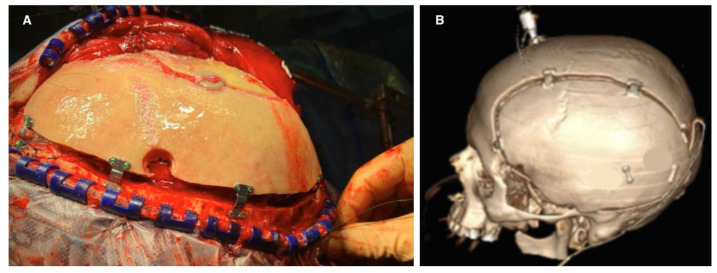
Panel (A) shows an intraoperative view of an expansion craniotomy with specialized fixation plates in place, demonstrating elevation of the bone flap above the cranial rim. Panel (B) presents a postoperative three-dimensional CT reconstruction confirming the maintained elevation of the bone flap and the appropriate positioning of the fixation hardware.
